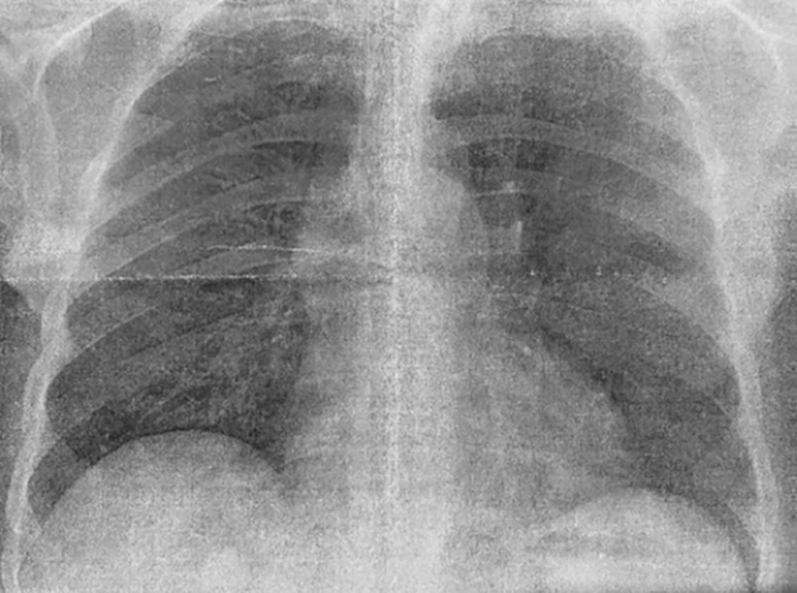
Chest X-Ray Demonstrating Mediastinal Widening in the Main Pulmonary Artery Region
